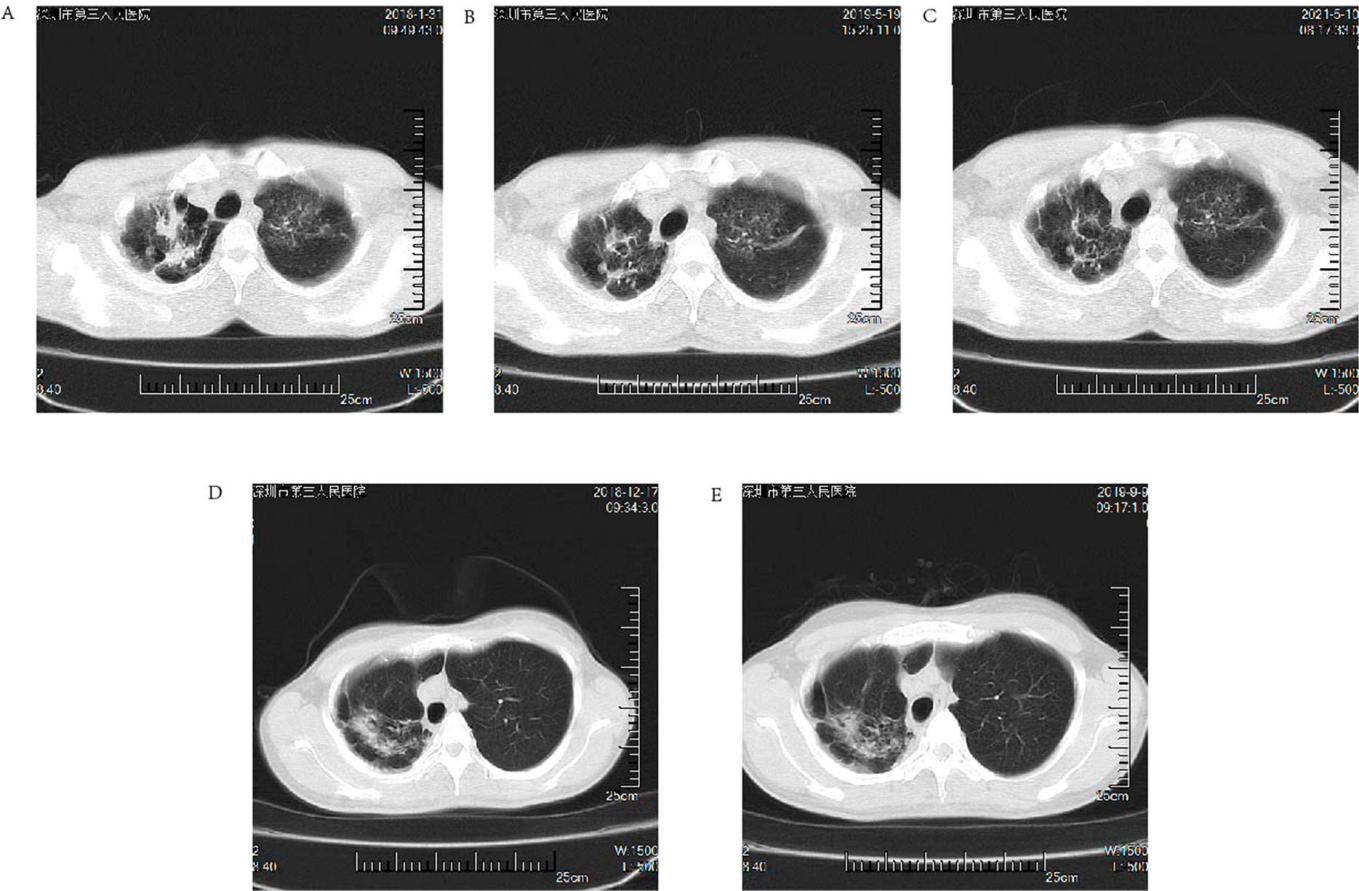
(A through E): Short-term follow-up HRCT images of patients with *Mycobacterium paragordonae* pulmonary disease; (A): HRCT images at the initial diagnosis in case 6; (B) HRCT images after the end of anti-tuberculosis treatment in case 6; (C) HRCT images 2 years after cessation of treatment in case 6; (D, E) HRCT images at the initial diagnosis and after 9 months of follow-up in case 8, respectively.
